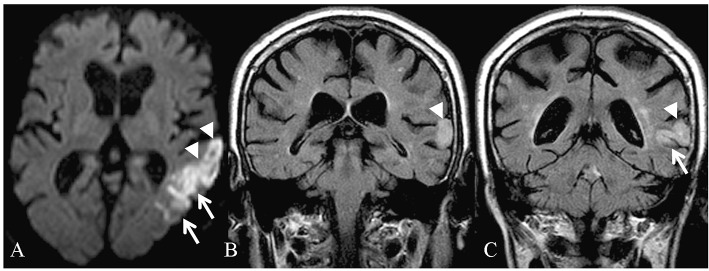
Magnetic resonance imaging of the patient 1 in the acute phase: restricted diffusion is identifiable in the left temporal lobe (A) on diffusion-weighted imaging (DWI). Coronal fluid-attenuated inversion recovery (FLAIR) images (B,C) demonstrate increased signal in the region of the superior temporal gyrus, on the left.
